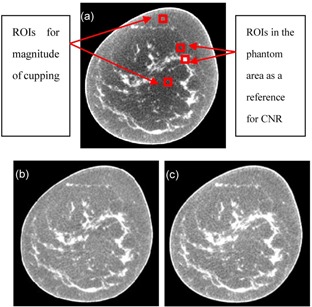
Comparison of breast CT images before (bottom left) and after (top) cupping artifact correction, using the method developed by Yang et al.^(19)^ (Bottom right) This paper's method.
